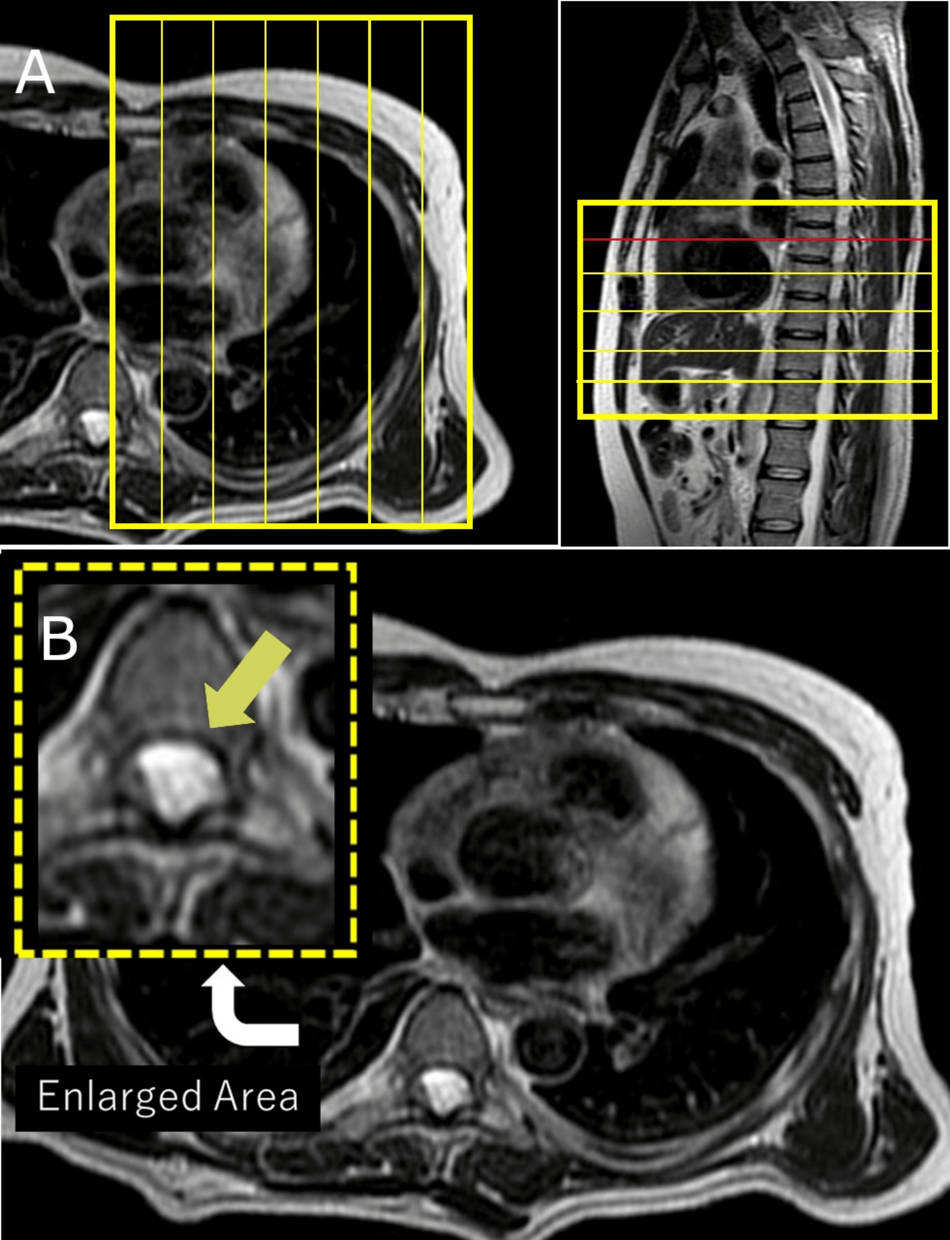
Initial chest MRI with a limited field of view (A) Initial chest MRI (sagittal and axial views) acquired at an external institution: the field of view (FOV) was focused primarily on the thoracic cavity, excluding adequate visualization of the spinal canal. In the sagittal image, the spinal canal and nerve roots fall outside the FOV, and only a single axial slice incidentally captures the lesion at the uppermost level. (B) Axial T2-weighted MRI at the T7 level: a faint hyperintense lesion is seen in the posterior aspect of the spinal canal. The inset highlights the unreported lesion (arrow), which was initially overlooked due to its peripheral location within the limited imaging field.
